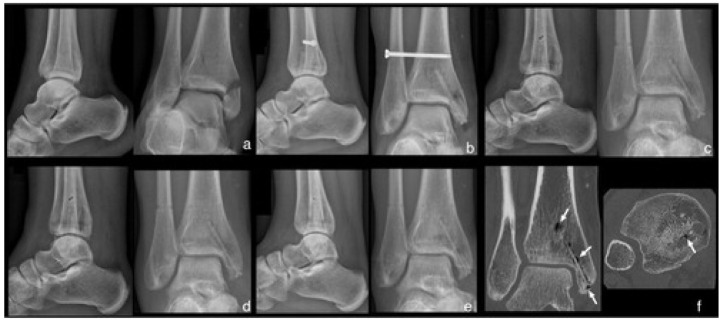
(a) Anteroposterior and lateral ankle radiographs of a 49-year-old female patient with medial malleolar fracture treated with Mg-based screws. (b) After 2 weeks: visible fracture line at the medial malleolus associated with small signs of radiolucent zones within the bone surrounding the screws. (c) After 6 weeks: fracture consolidation with increase of radiolucent zones within the bone surrounding the screws. (d) After 12 weeks, and (e) After 24 weeks: constant and non-evolutive radiolucent zones. (f) CT scan at 52 weeks, decrease of radiolucent zones, increased endosteal bone mass, and periosteal bone ingrowth at the screw head (white arrow). Reproduced from [108].
